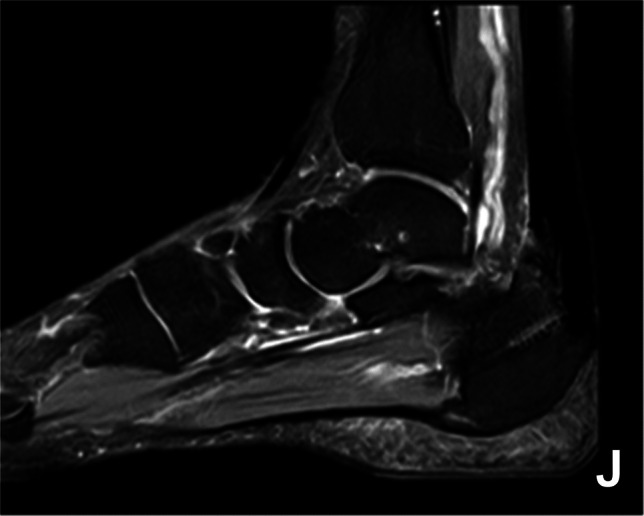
MRI images at 12 months postoperatively demonstrated that the continuity of the Achilles tendon has been reconstructed
